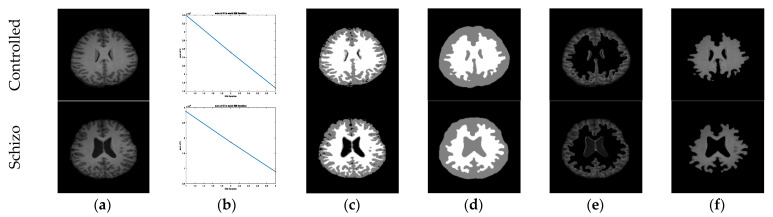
Separation of brain MRI slice into GM and WM: (a) Image; (b) Convergence; (c) Initial; (d) Final; (e) GM; (f) WM.
